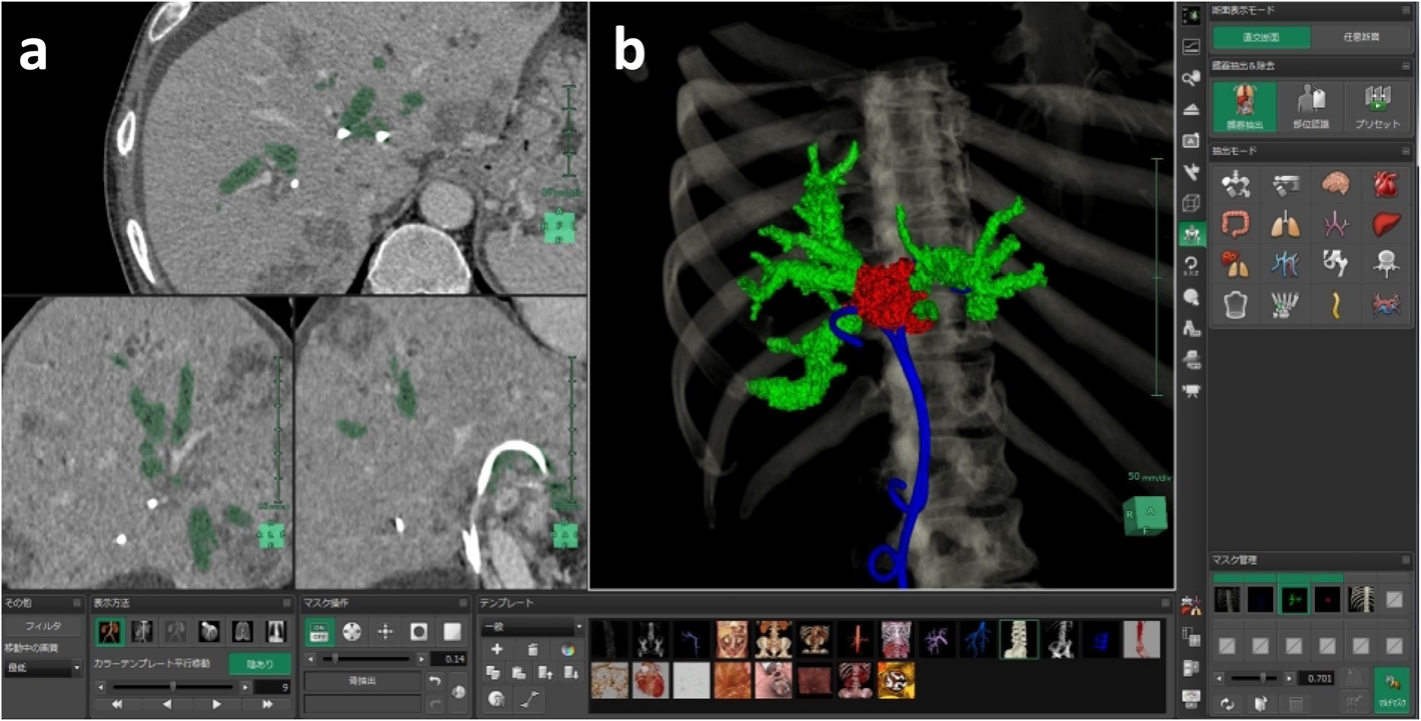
Creation of 3D images of the biliary tree from CT image data using a 3D image analysis system (SYNAPSE VINCENT version 5, FUJIFILM Corporation, Tokyo, Japan). a: CT images. b. A 3D image created by tracing dilated intrahepatic bile ducts. Green: dilated bile ducts. Red: metastatic liver tumor at hilar biliary region. Blue: PS tubes placed previously. CT, computed tomography; PS, plastic stent; 3D, three-dimensional
